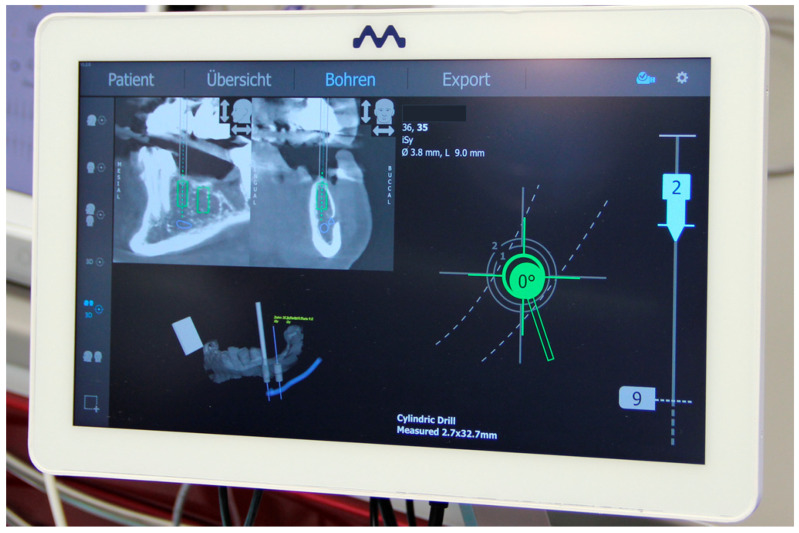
Real-time representation of the drill position (the position, angle, and depth) as well as a sectional view of the planned implant position in the CBCT.
